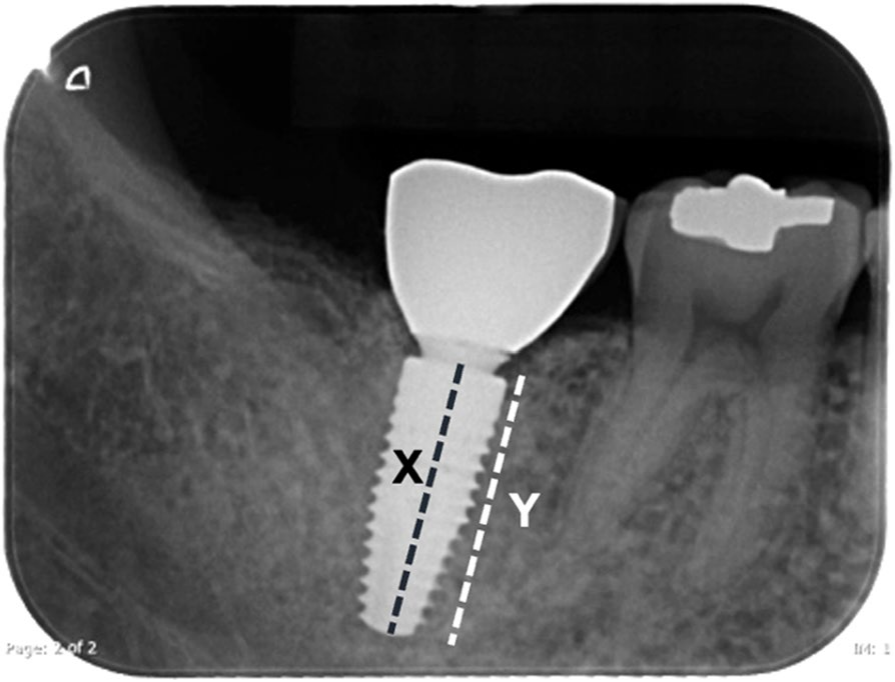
Measurement of X and Y parameters to assess marginal bone change from the periapical radiograph
